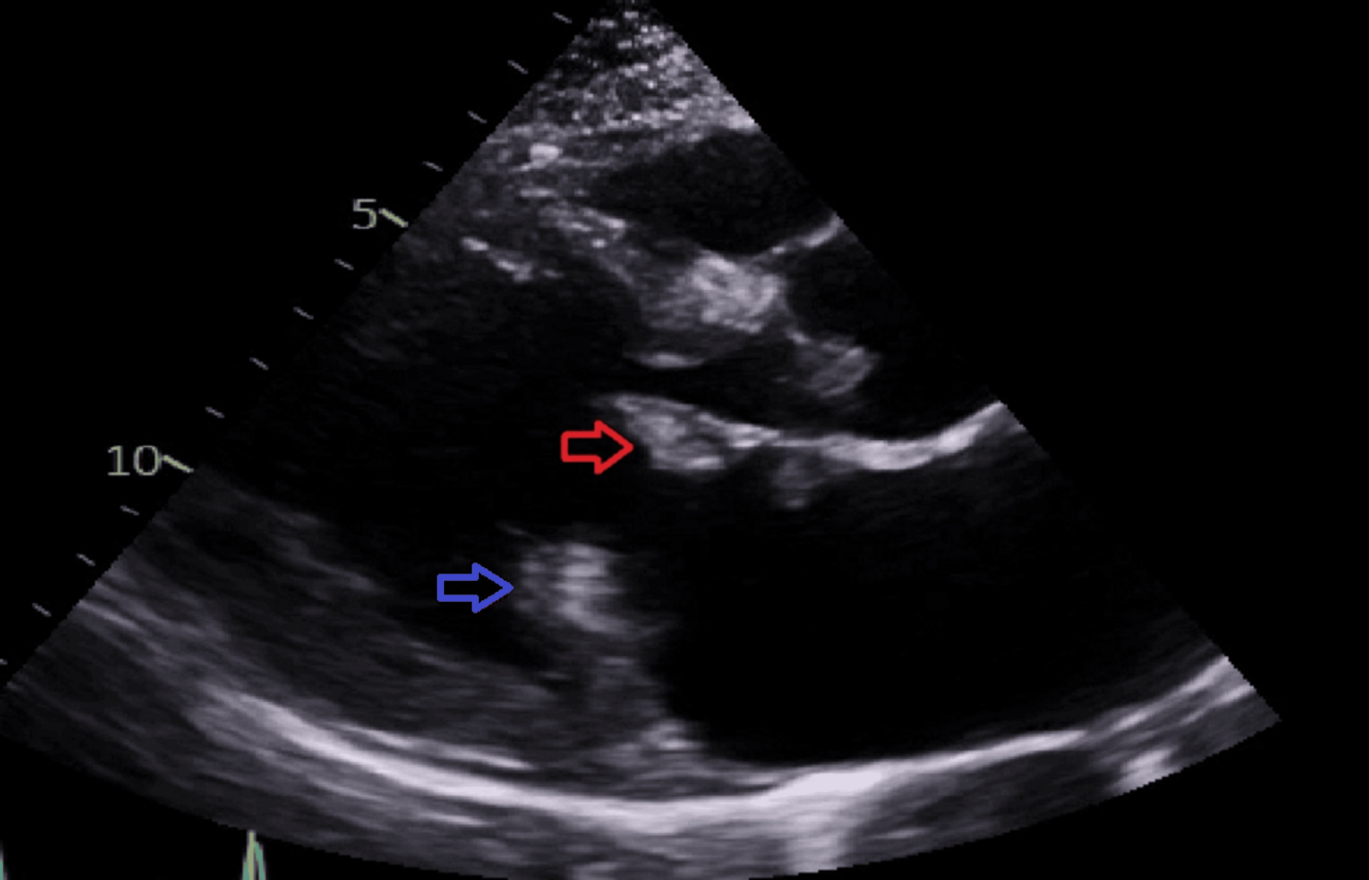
Echocardiogram showing PLAX view of the heart A large (1.4 × 2.1 cm), mobile mass attached to the mid-portion of the atrial surface of the anterior mitral valve leaflet is seen (red arrow). A smaller, mobile mass on the tip of the posterior mitral valve leaflet is also noted (blue arrow). PLAX: parasternal long-axis
